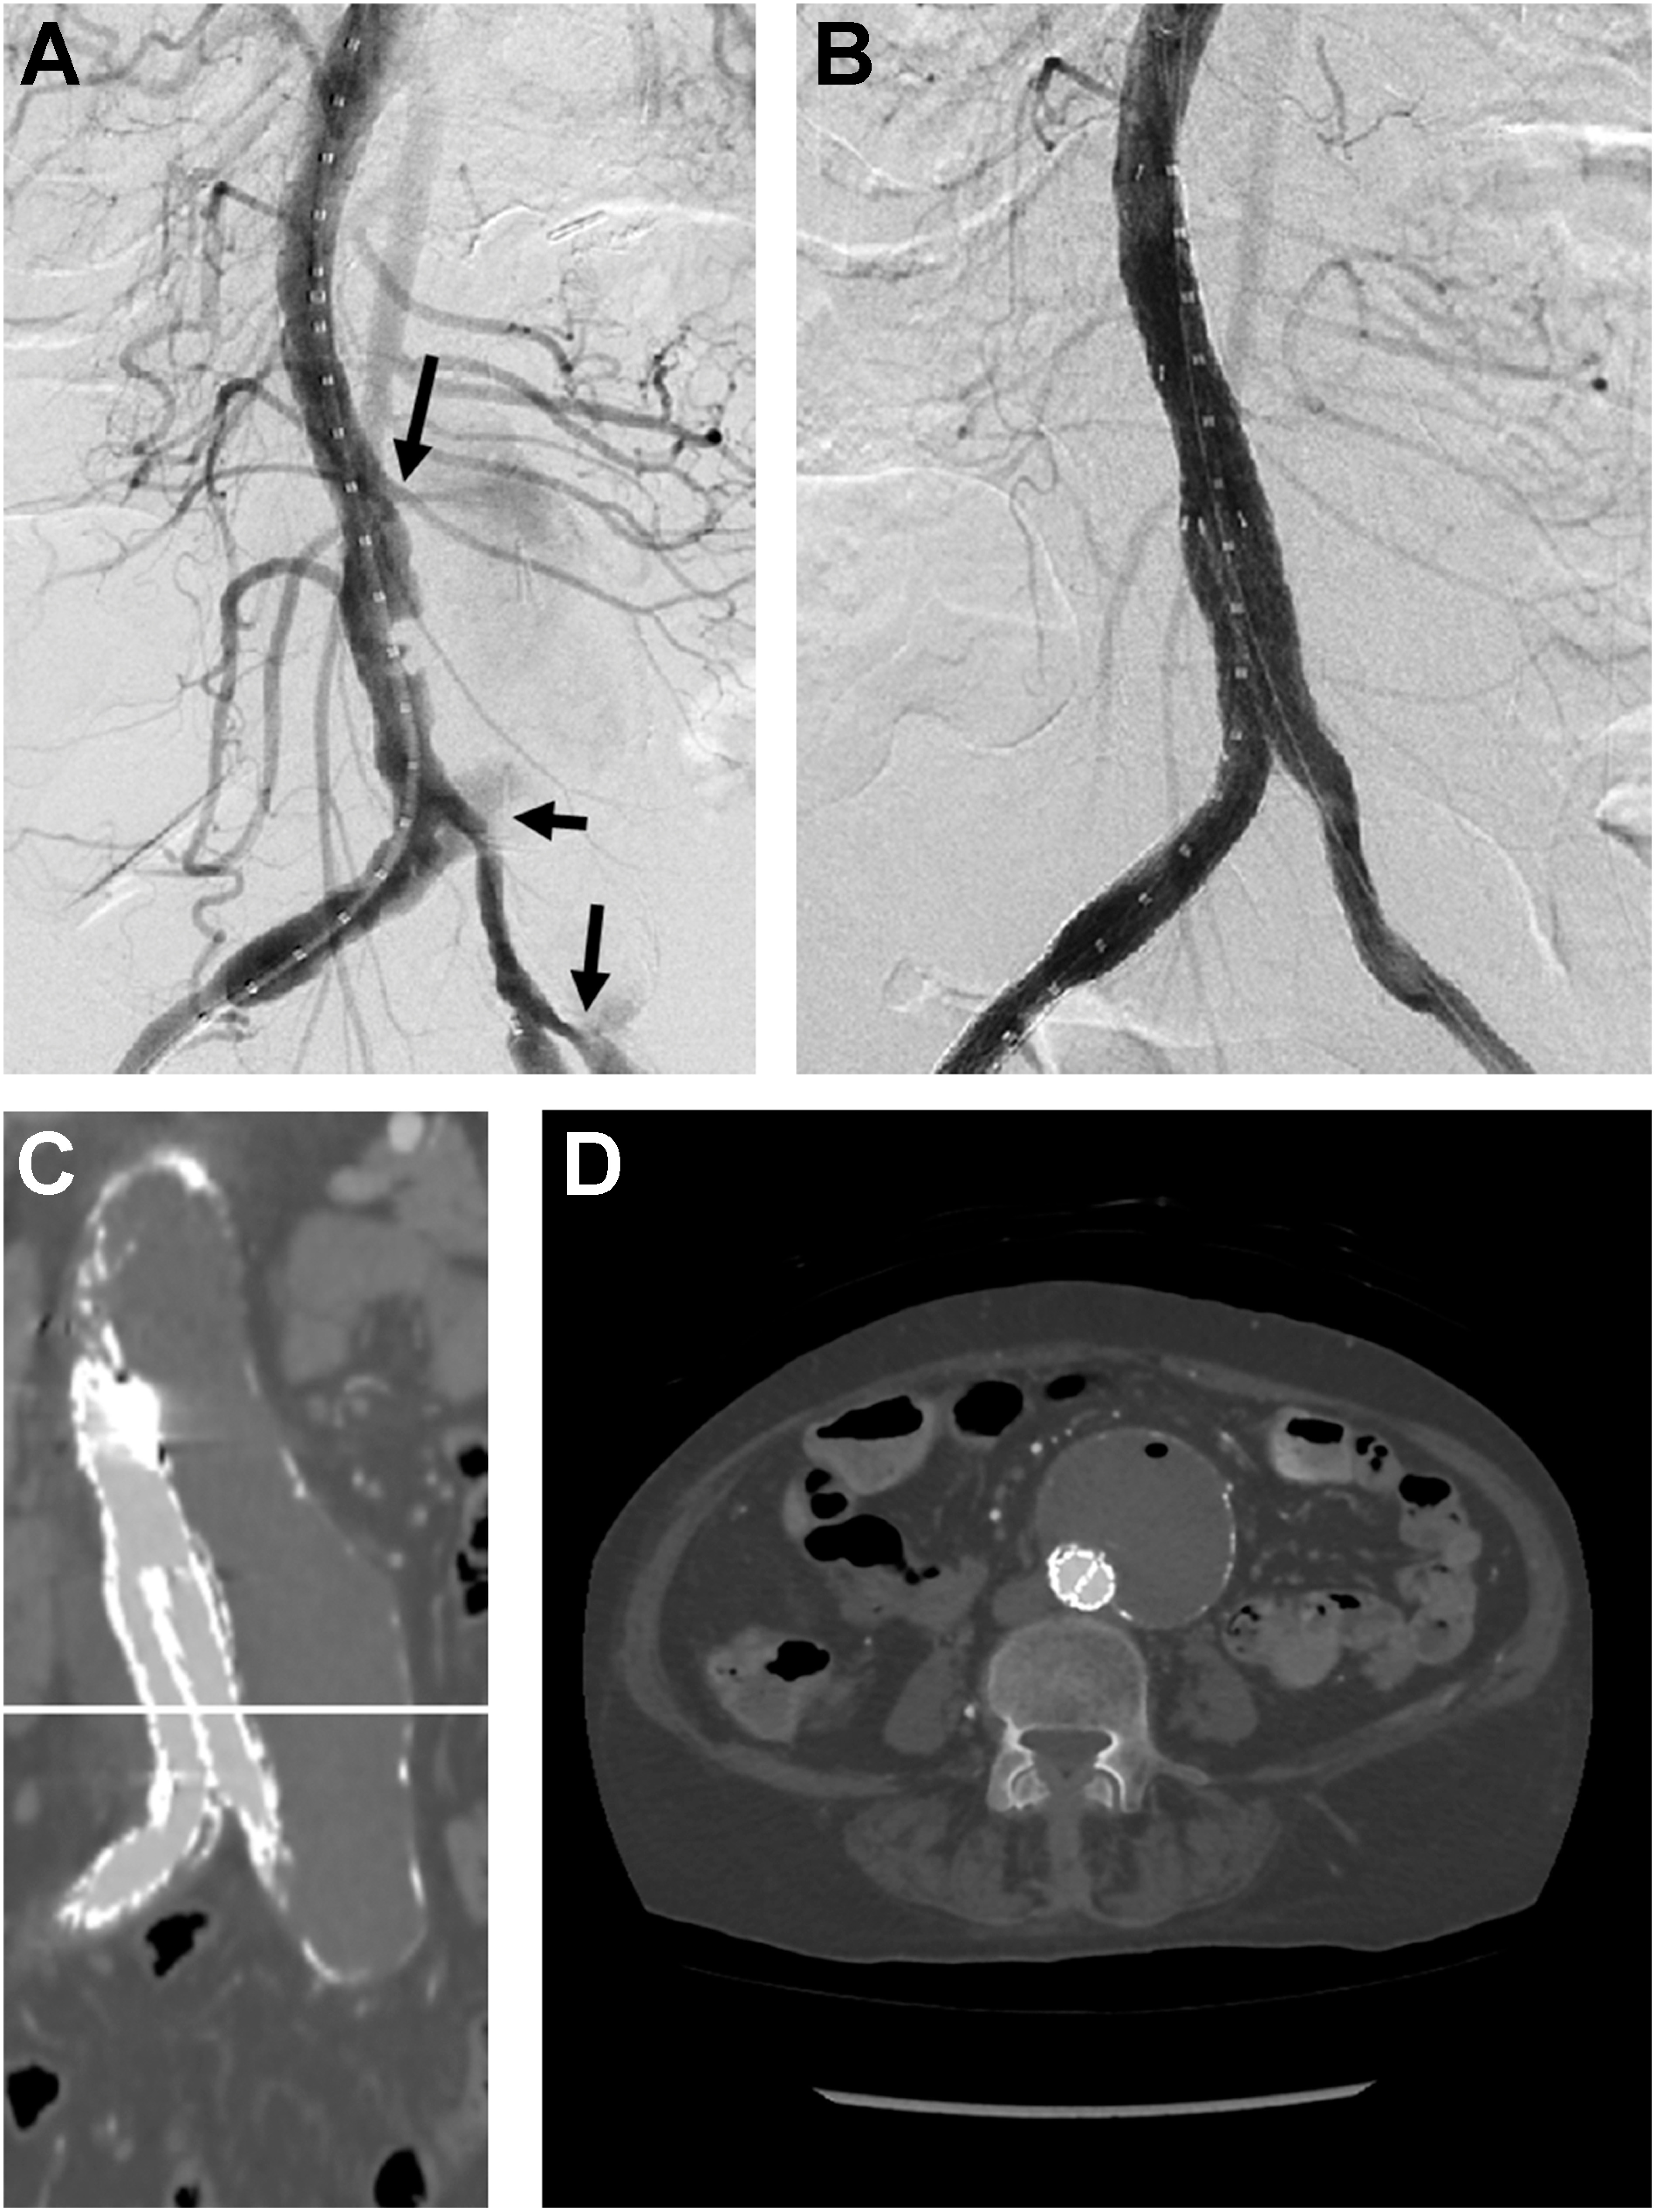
Fig. 2 (A) The initial angiogram shows three communications between the true and false lumen (arrows). (B) The completion angiogram shows no endoleak. (C, D) Multi-planar reconstruction image of postoperative computed tomography shows parallel configuration of Gore Excluder legs. The white horizontal line shows the level of the axial image (D). (D) The axial image shows no endoleak or collapse of legs.
